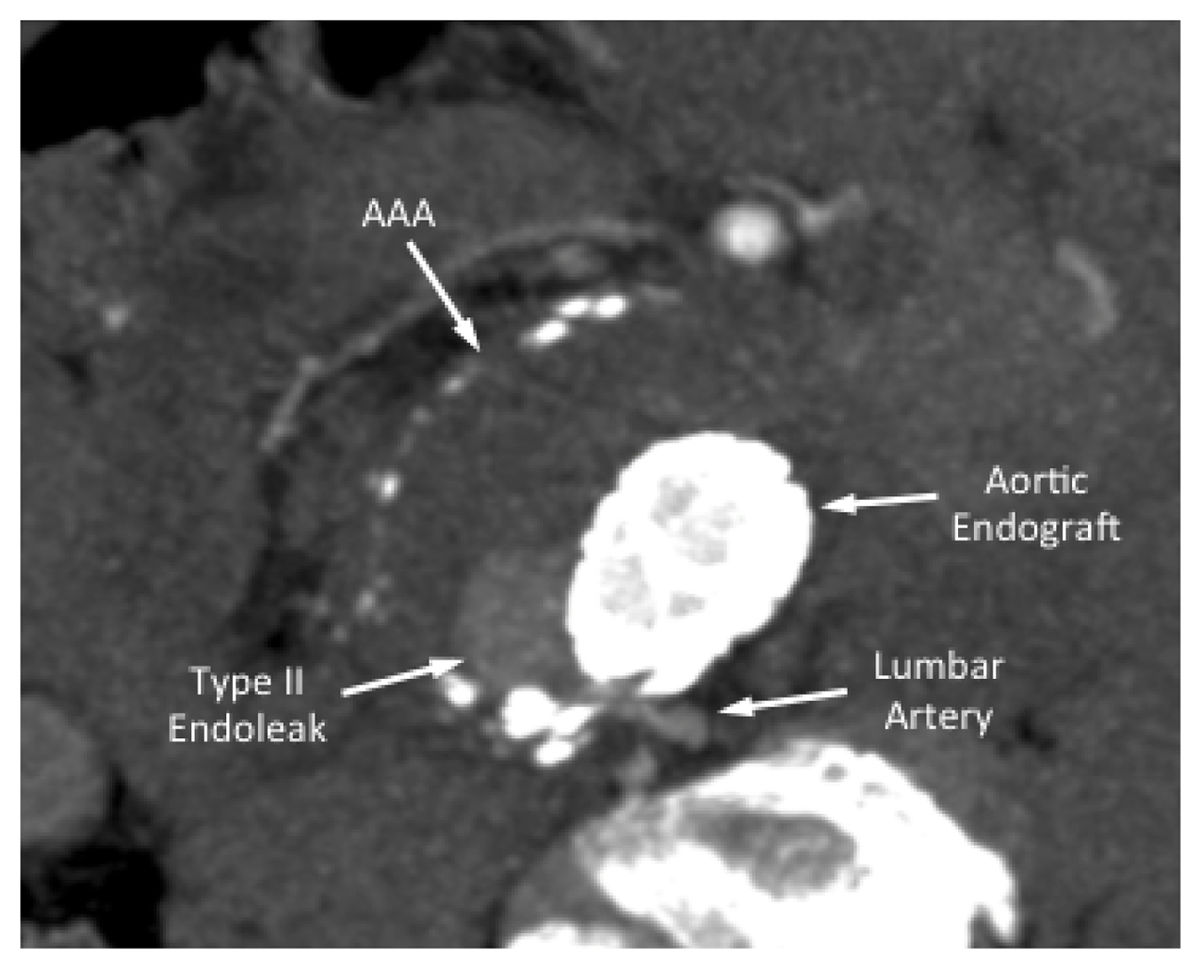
Type II endoleak from a lumbar artery. Following EVAR, follow-up CTA demonstrated delayed focal aortic aneurysm sac filling from a posterior aortic lumbar artery branch.
